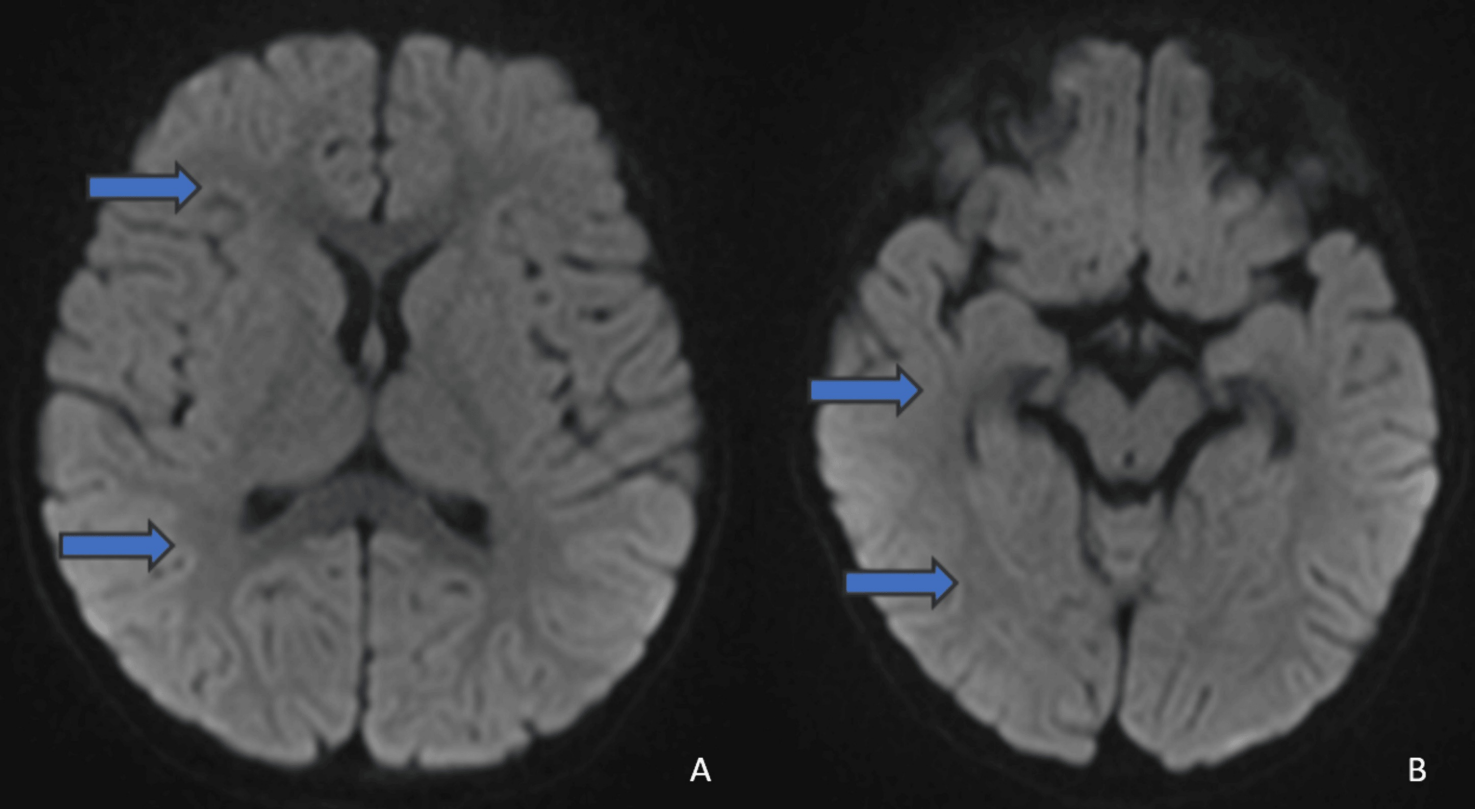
Diffusion-weighted images on day 14 show no high signal on DWI images in bilateral frontal and parietal lobes [4A] as well as bilateral temporal and occipital lobes [4B]. DWI: Diffusion weighted imaging
